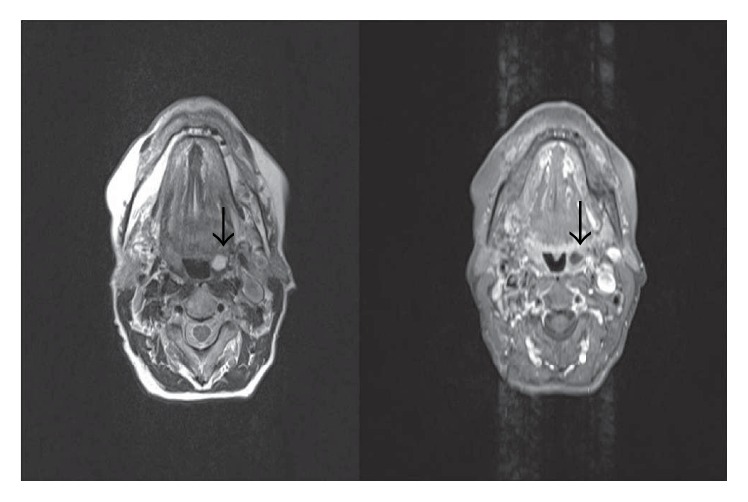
A cystic mass (black arrow) hypointense on T1 images and hyperintense on T2 was observed inside the right tonsil at magnetic resonance imaging (MRI).
